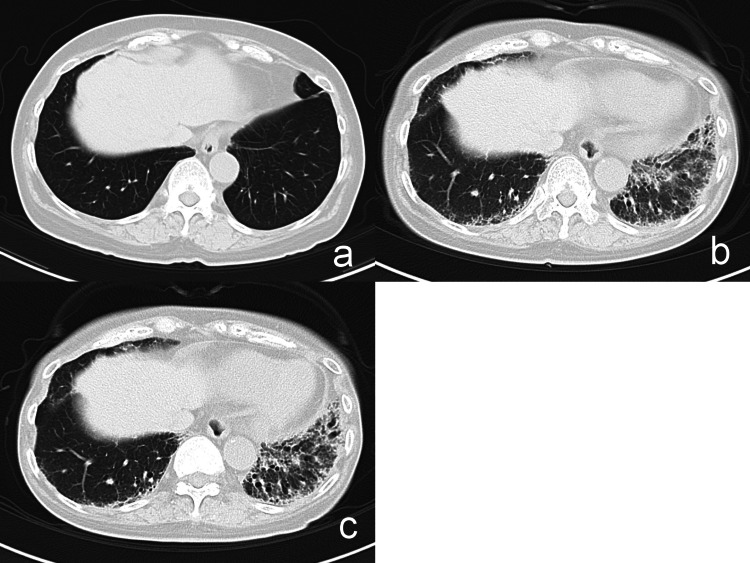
Chest computed tomography a: CT images in August 2017 reveal no noteworthy pulmonary lesions, including interstitial alterations. b: Before treatment, ground-glass opacities, reticular patterns, and traction bronchiectasis were discernible in both lower lung lobes, predominantly on the left side. c: After two months of treatment with mycophenolate mofetil, no considerable alterations were detected in the interstitial pattern in either lung.
